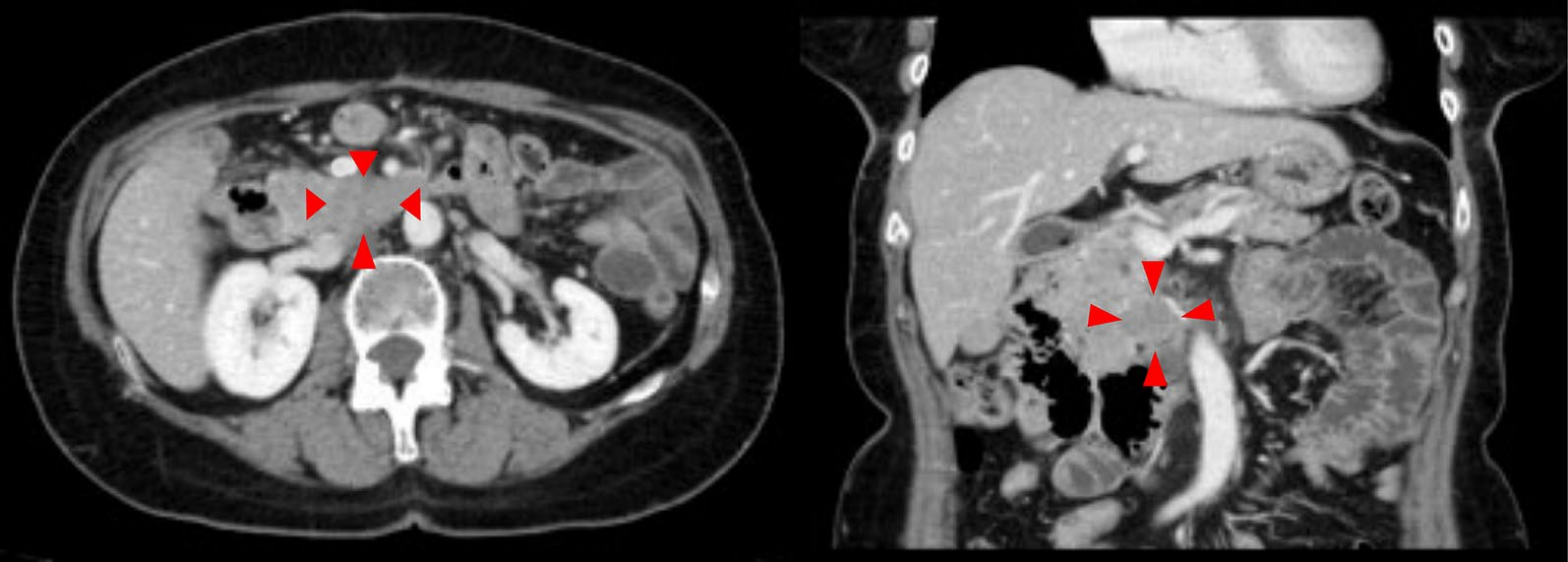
CT at the time of recurrence. Lymph node enlargement was detected in the dorsum of the pancreatic uncus 10 months after the surgery. CT, computed tomography
